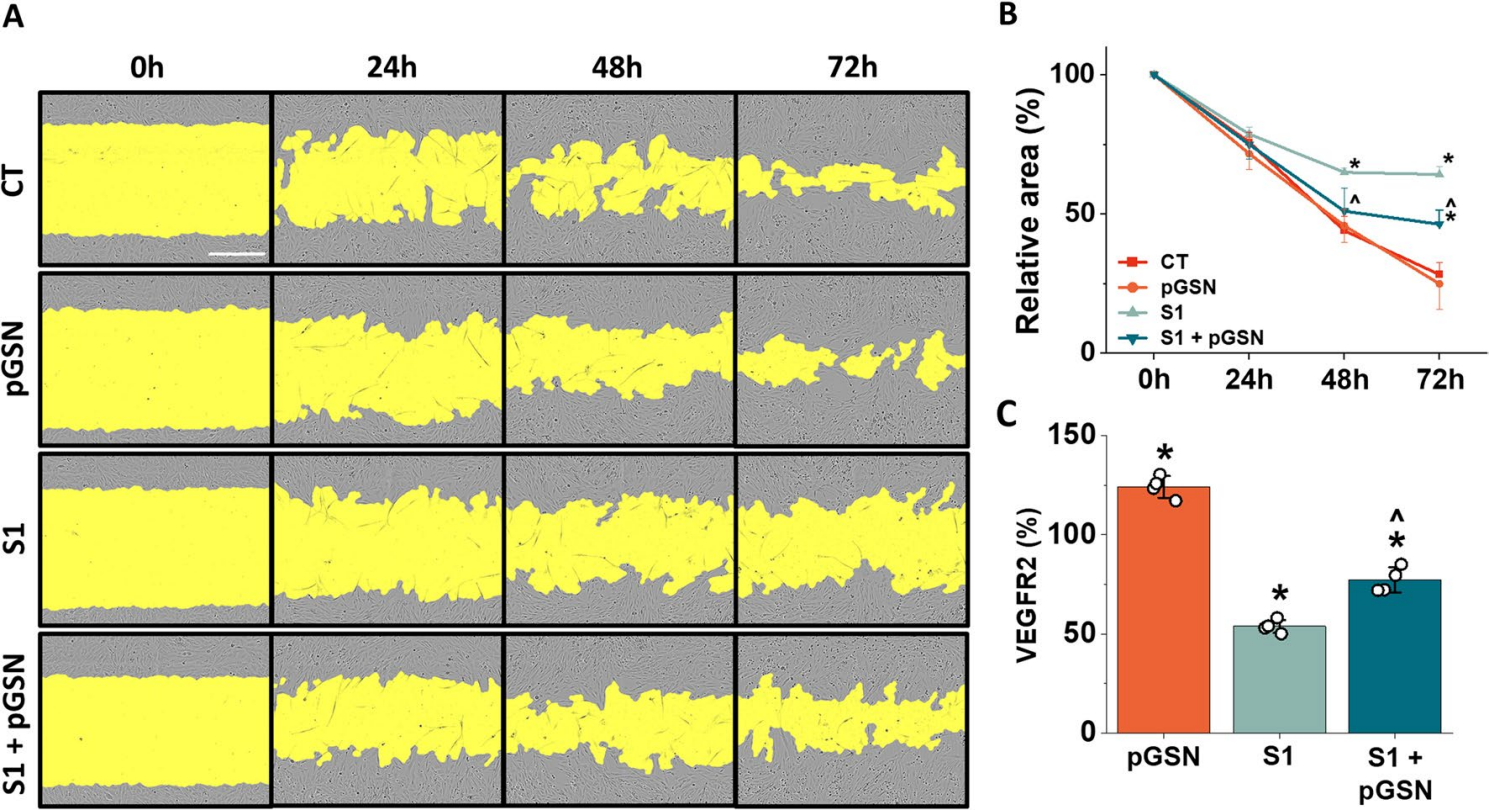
Plasma gelsolin improves migration of hCMEC/D3 upon SARS-CoV-2 Spike protein S1 subunit treatment in wound-healing assay. Images of endothelial cells in a wound healing setting (Panel A), the yellow color indicates wound width, which is quantitatively shown in Panel B. Western blot quantitative analysis of VEGRF2 expression in hCMEC/D3 cells after 24 h stimulation with pGSN, S1, and S1 + pGSN (Panel C). The data represent the mean ± SEM of four independent experiments (*n* = 4). * and ^ indicate statistical significance at *p* ≤ 0.05 compared to CT (100%) and S1, respectively, by one-way ANOVA and Tukey post hoc test
